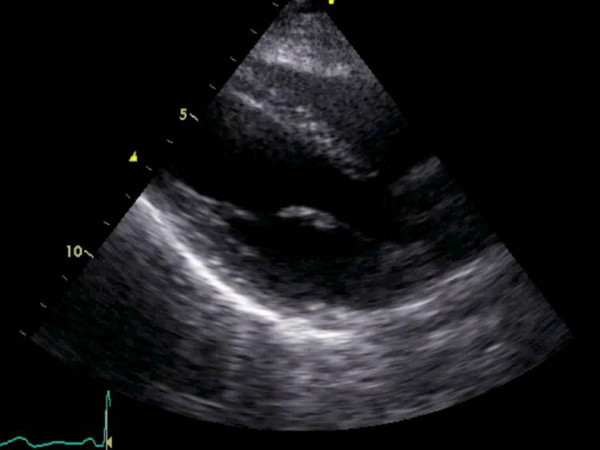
In order to improve the acoustic window, the parasternal long axis view was modified by the echocardiographer causing tilting of the image.
